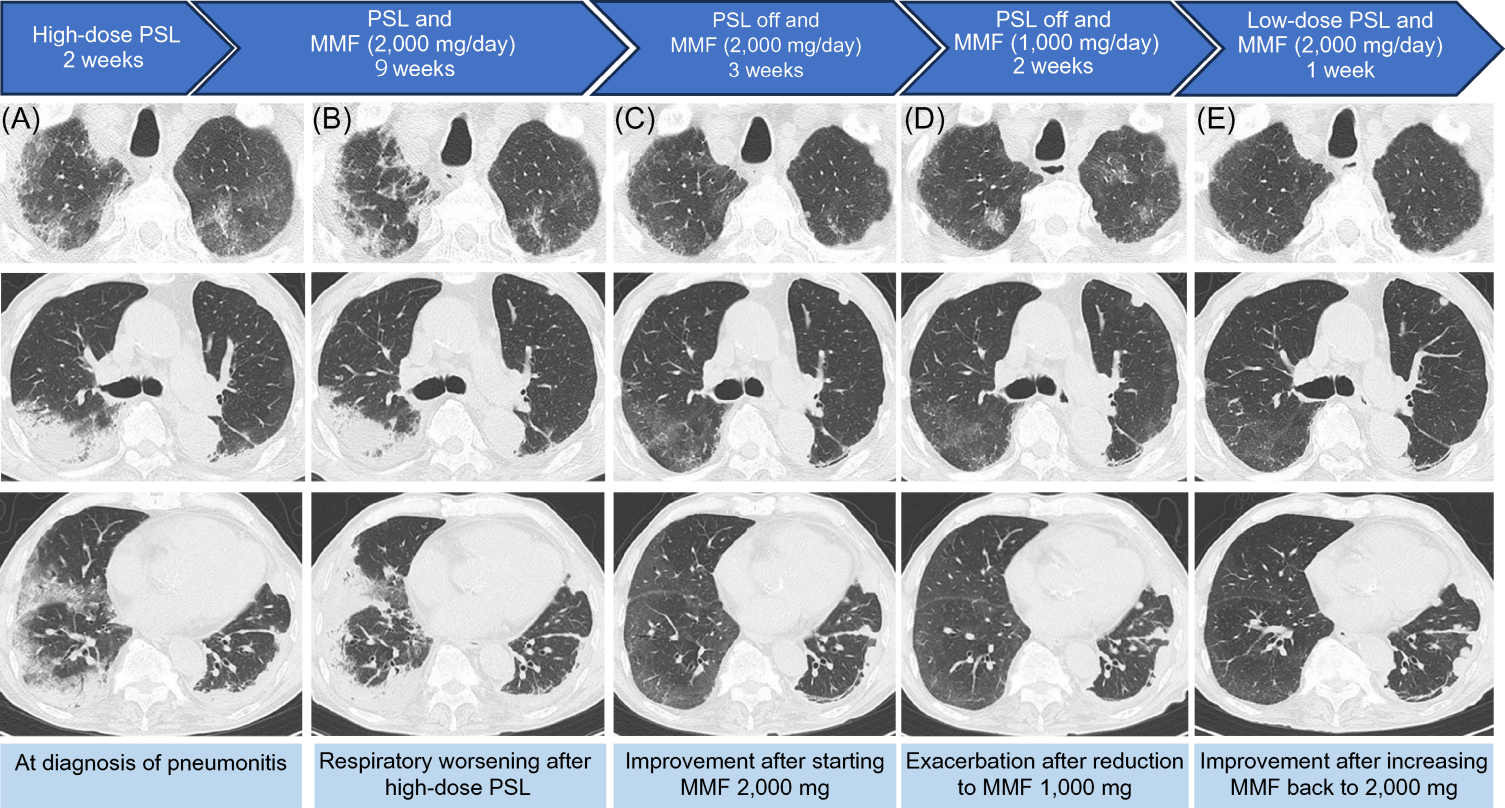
Treatment details and duration for pneumonitis with changes in chest computed tomography are presented (A) at the time of pneumonitis diagnosis; (B) at time of respiratory worsening after high‐dose PSL; (C) start of MMF 2000 mg showing some improvement; (D) after reduction to MMF 1000 mg showing exacerbation of symptoms; and (E) after increasing the dose back to 2000 mg showing improvement. MMF, mycophenolate mofetil; PSL, prednisolone.
